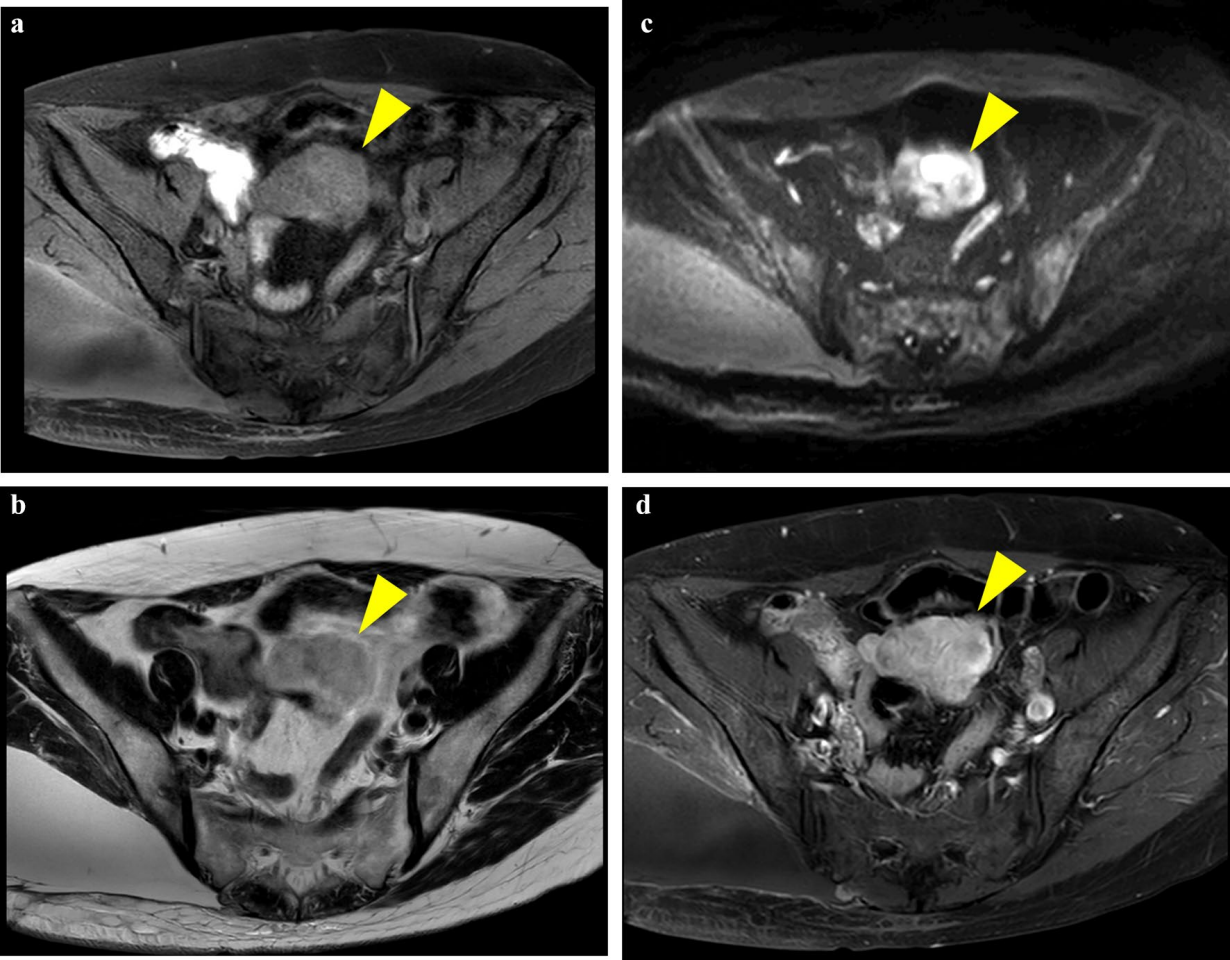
Magnetic resonance imaging showing a lobulated mass with a low intensity on T1-weighted and b high intensity on T2-weighted images. c Magnetic resonance imaging diffusion-weighted images showing diffusion restriction of the mass. d Contrast-enhanced magnetic resonance imaging showing contrast enhancement of the mass
